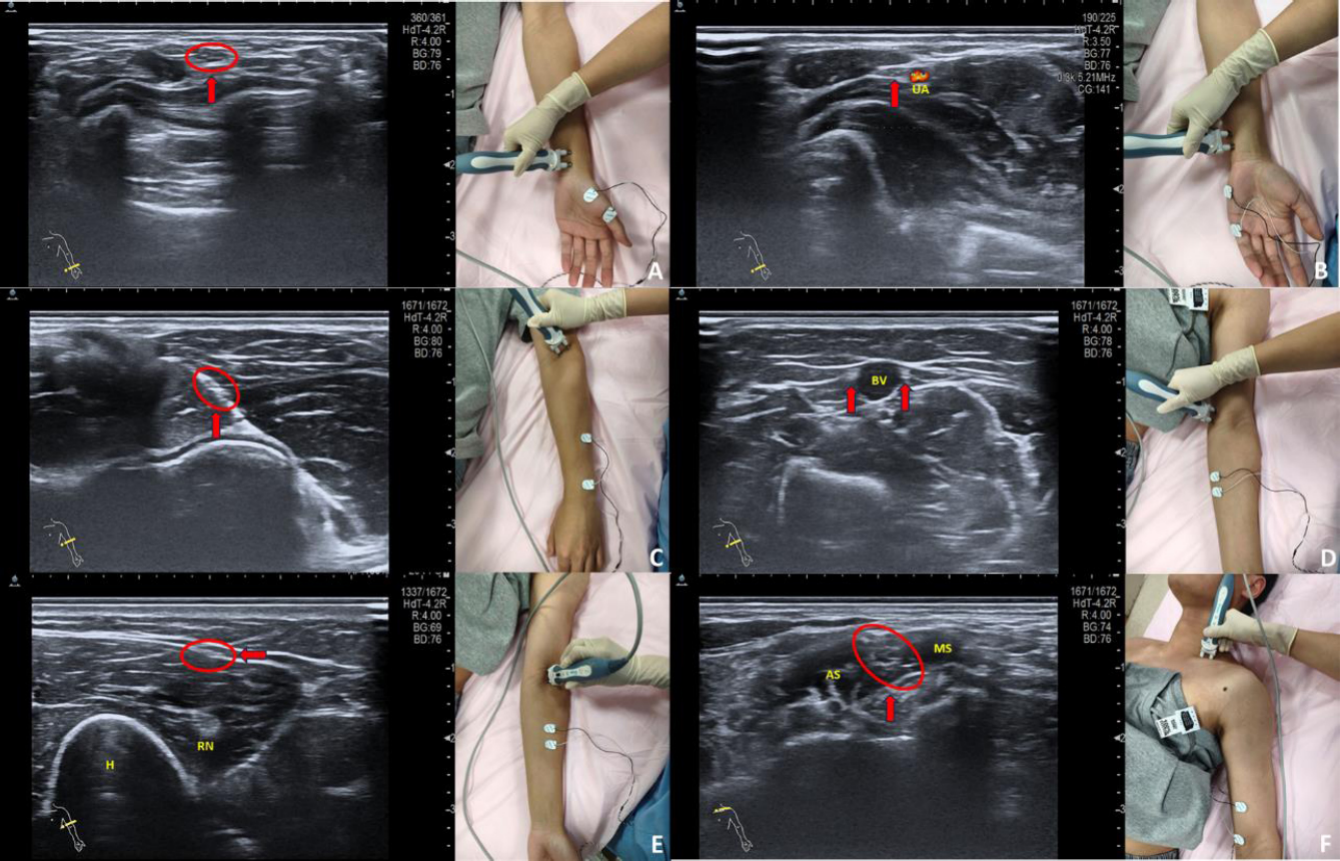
Localization and examination workflow of partial brachial plexus branches. (A) Left: Short-axis ultrasound image of median nerve (red arrow) showing its localization; Right: Nerve conduction study of median nerve with the stimulating electrode placed at the identified location. (B) Left: Short-axis ultrasound image of ulnar nerve (red arrow) located adjacent to the ulnar artery(UA); Right: Nerve conduction study of ulnar nerve with the stimulating electrode placed at the identified location. (C) Left: Short-axis ultrasound image of radial nerve (red arrow) showing its localization; Right: Nerve conduction study of radial nerve with the stimulating electrode placed at the identified location. (D) Left: Short-axis ultrasound image of medial antebrachial cutaneous nerve (red arrow) located adjacent to the basilic vein (BV); Right: Nerve conduction study of medial antebrachial cutaneous nerve with the stimulating electrode placed at the identified location.(E) Left: Short-axis ultrasound image of lateral antebrachial cutaneous nerve (red arrow) located superficial to the radial nerve (RN) and humerus (H); Right: Nerve conduction study of lateral antebrachial cutaneous nerve with the stimulating electrode placed at the identified location. (F) Left: Ultrasound image of interscalene brachial plexus (red arrow), positioned between the anterior scalene (AS) and middle scalene (MS); Right: Nerve conduction study of musculocutaneous nerve with the stimulating electrode placed at Erb’s point.
